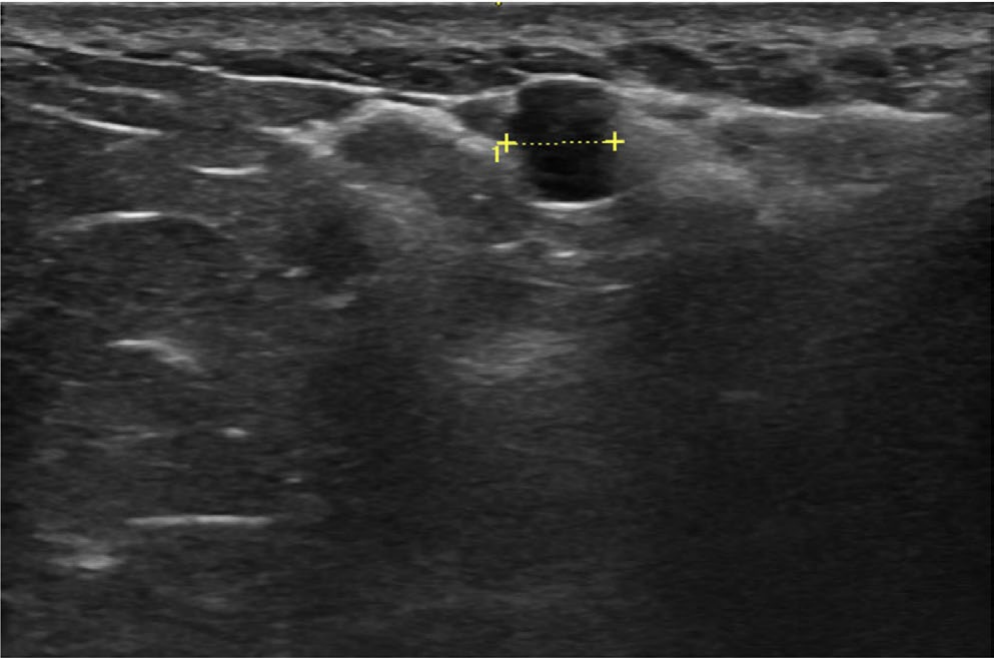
Liquid silicone. Ultrasound images showing infiltrative echogenic silicone oil in the epidermis, producing a characteristic “snowstorm” artifact. Anechoic vesicles, taller than wide, are interspersed within the filler, with a posterior echogenic linear artifact indicative of pure silicone.
